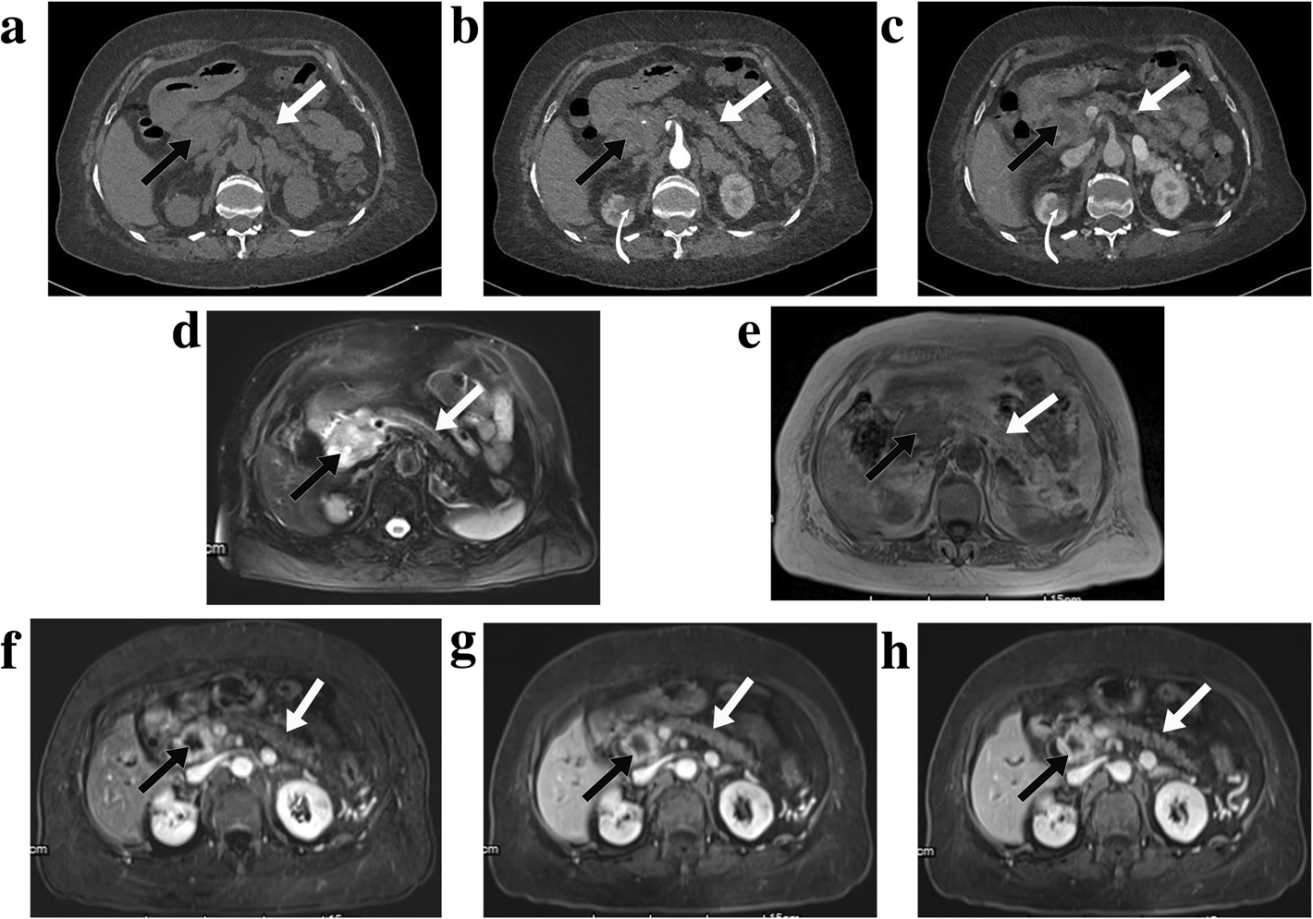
Type 1 autoimmune pancreatitis with concurrent pancreatic ductal adenocarcinoma. Axial pre-enhanced (a), arterial (b), venous (c) phase on CT and axial T2WI (d), T1WI (e), pancreatic (f), portal venous (g) and delayed venous (h) phase on MR imaging showed a focal lesion in the pancreatic head (straight black arrows). It appeared as low-density on enhanced CT images (b and c). The imaging characteristic of rim enhancement was noted on enhanced MR images (f, g and h), which was not obvious on CT. Distal pancreatic parenchymal atrophy was observed (straight white arrows on a-h). Kidney involvement was also seen (curved white arrows on b and c)
